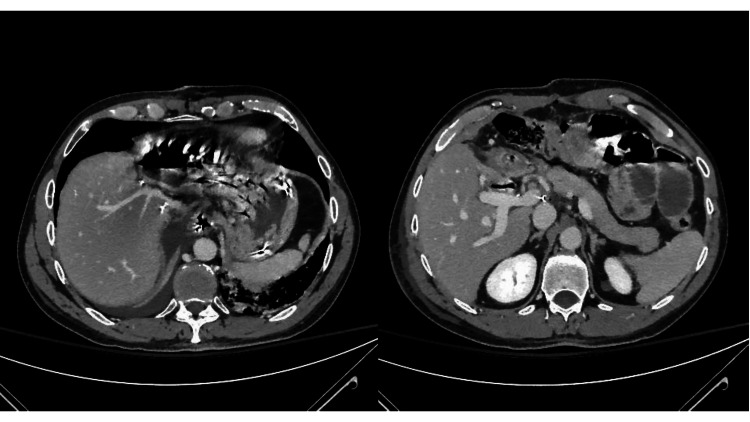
Postoperative CT scan four days after surgery.
